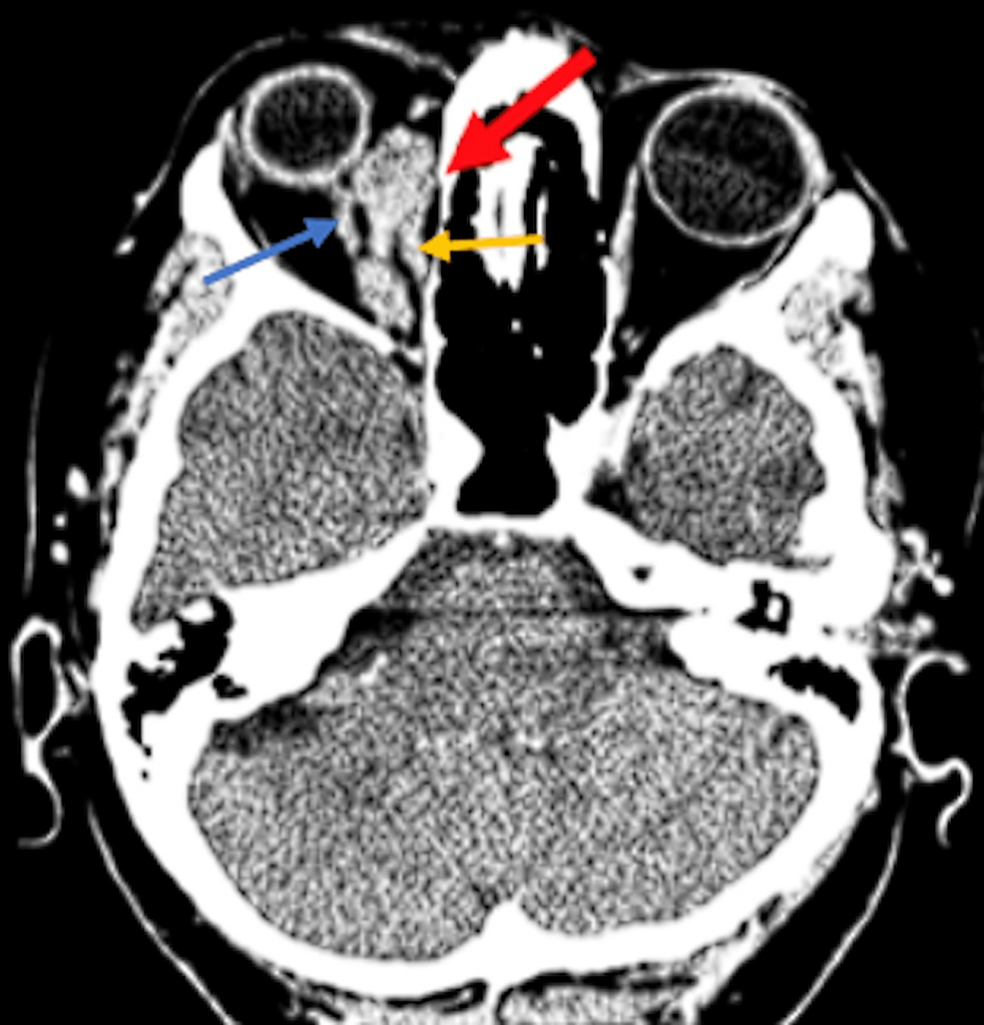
Axial CT of the patient during admission. Of note is the lobulated enhancing soft tissue mass lesion (red arrows) in the superomedial aspect of the right orbit with involvement of the right optic nerve (blue arrow) and the medial rectus muscle (yellow arrow) leading to mild proptosis. CT: computed tomography
